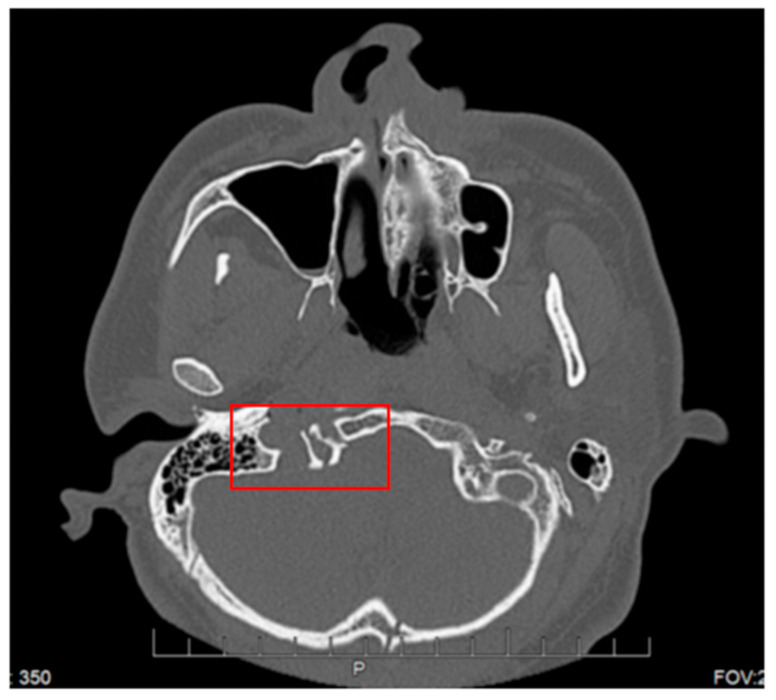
CT maxillofacial. Red box indicates junction of Jugular foramen and Hypoglossal Canal.
